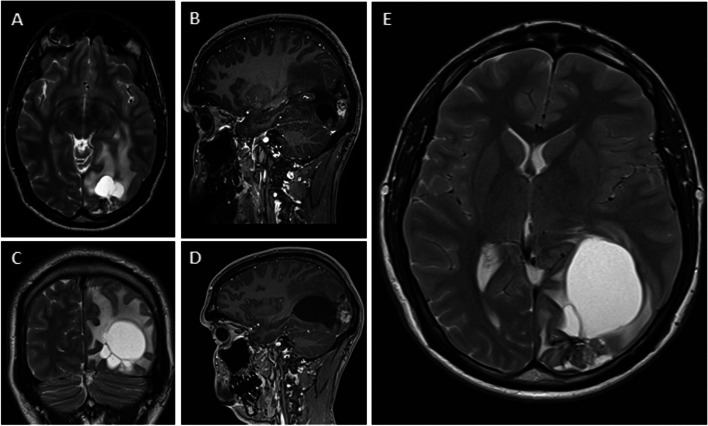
Serial MRI after AVM treatment with SRS showing an enlarging left occipital cystic formation with mass effect and edema. A Axial T2-weighted and B sagittal gadolinium-enhanced T1-weighted MRI 10 years after SRS treatment showed a cystic formation (11 × 16 × 15 mm) surrounded by vasogenic edema, adjacent to a contrast-enhancing angiomatous nodule. C Coronal T2-weighted, D sagittal gadolinium-enhanced T1-weighted, E axial T2-weighted MRI 1 year later (11 years post-SRS treatment) showing a significant enlargement of the cystic formation (70 × 35 × 50 mm), now multiloculated, and of the associated vasogenic edema with contralateral midline shift and incipient subfalcine herniation. The contrast-enhancing angiomatous nodule persists
